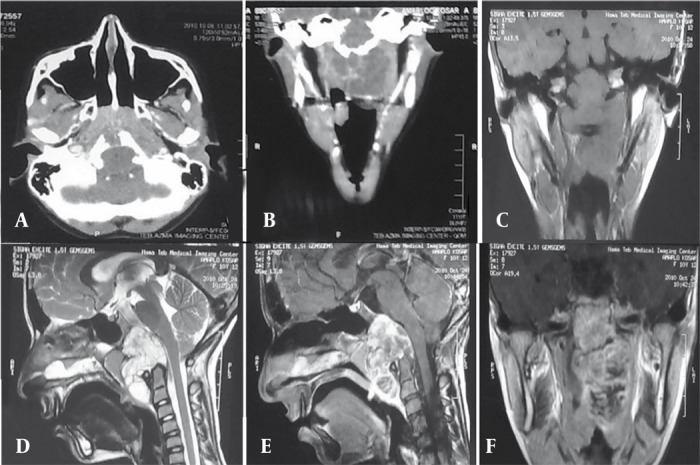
A and B, Contrast CT Scan Reveals a Heterogeneous Enhancing Mass in the Nasopharyngeal Region With Destruction of the Posterior Portion of Clivus and Bulging to Foramen Magnum . C-F, MRI With and Without Contrast Shows a Large Heterogeneous Signal Enhancing Mass in the Retropharyngeal Space of the Nasopharynx Extending Anteriorly to the Uvula and Posteriorly to Foramen Magnum Compressing the Medulla and Cord With Involvement of the Posterior Portion of the Clivus.
